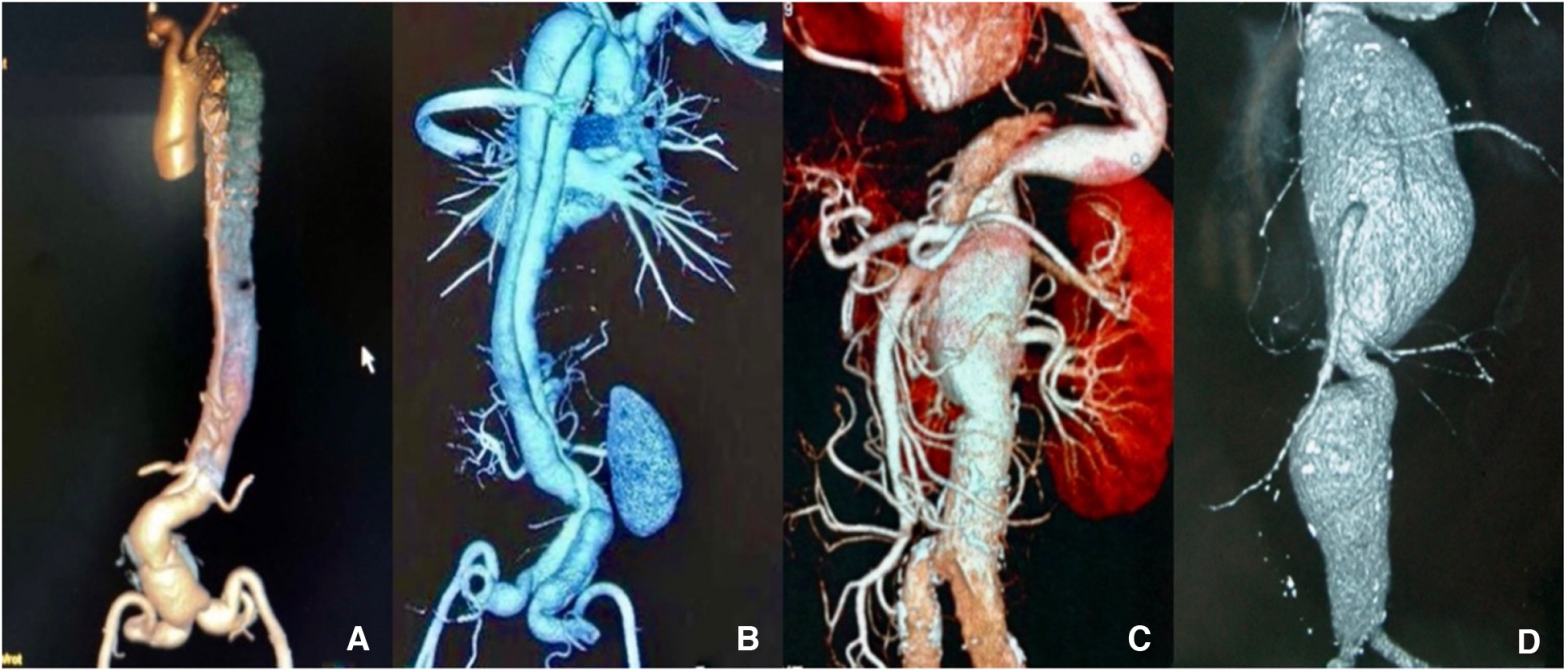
(A,B) Preoperative computed tomography angiography (CTA) scan shows thoracoabdominal aortic dissection (TAAD). (C,D) CTA shows thoracoabdominal aortic aneurysm (TAAA). The images show that all lesions of the aorta involve the visceral arteries.
